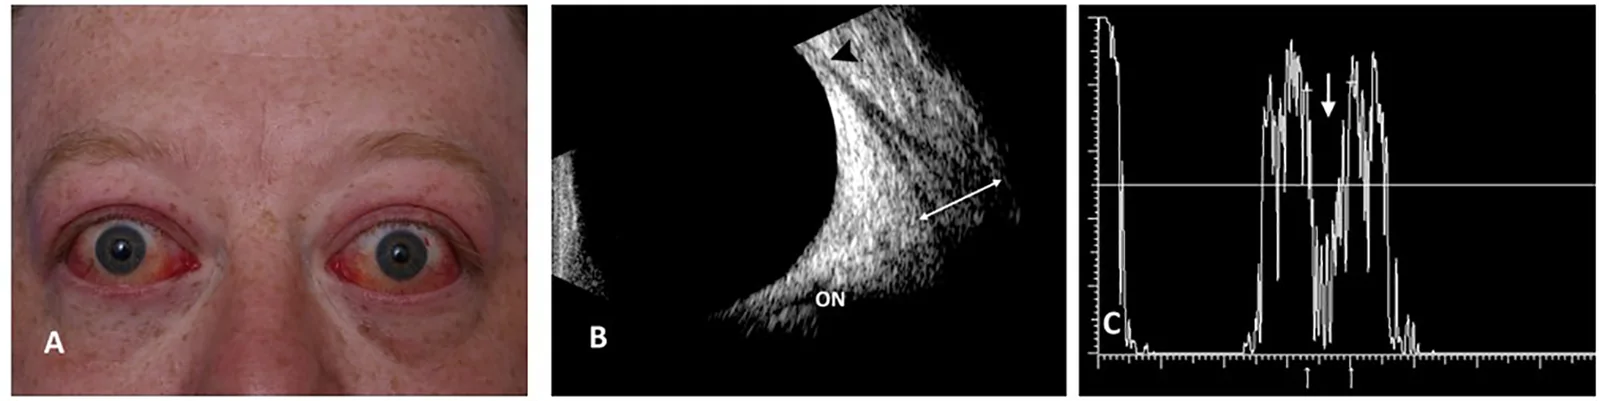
Thyroid Eye Disease. (A) External photography shows bilateral proptosis, upper and lower lid retraction, and injected conjunctiva. (B) Longitudinal B-scan of the right inferior rectus shows an enlarged muscle belly (double arrow) and normal muscle tendon (arrowhead). (C) Corresponding A-scan ultrasound of the right inferior muscle demonstrates low internal reflectivity. (arrow). *ON*, optic nerve.
